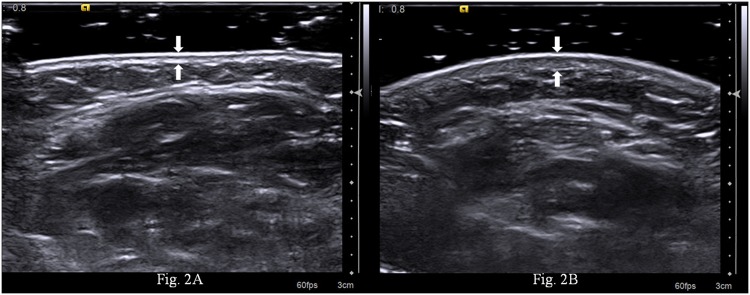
Ultrasound measured skin thickness of the dcSSc patients and the control’s right forearm. The skin thickness was 0.6mm (between arrows) for controls (Fig 2A), and 1.0mm (between arrows) for patients (Fig 2B).
